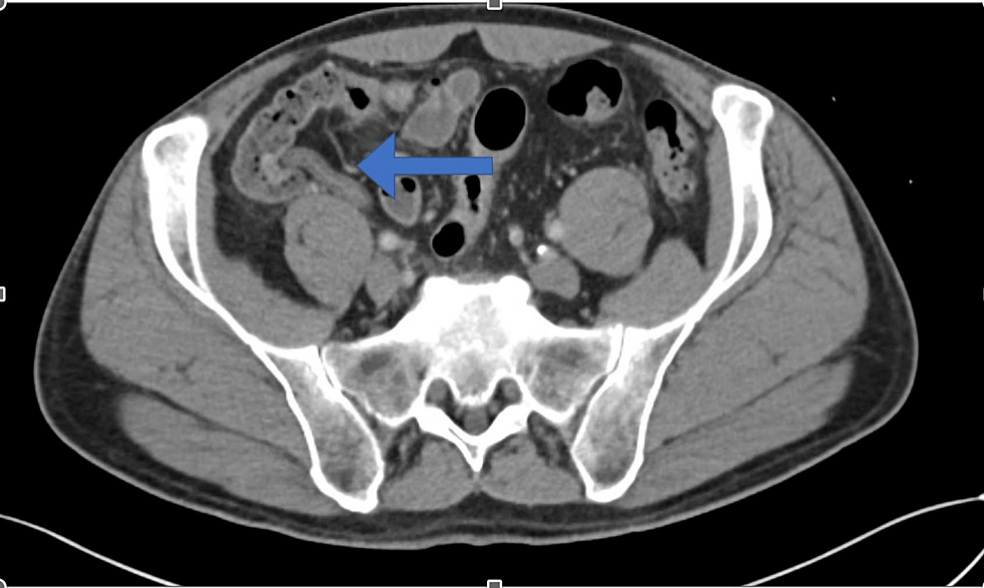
Axial computed tomography scan done on June 12th.
